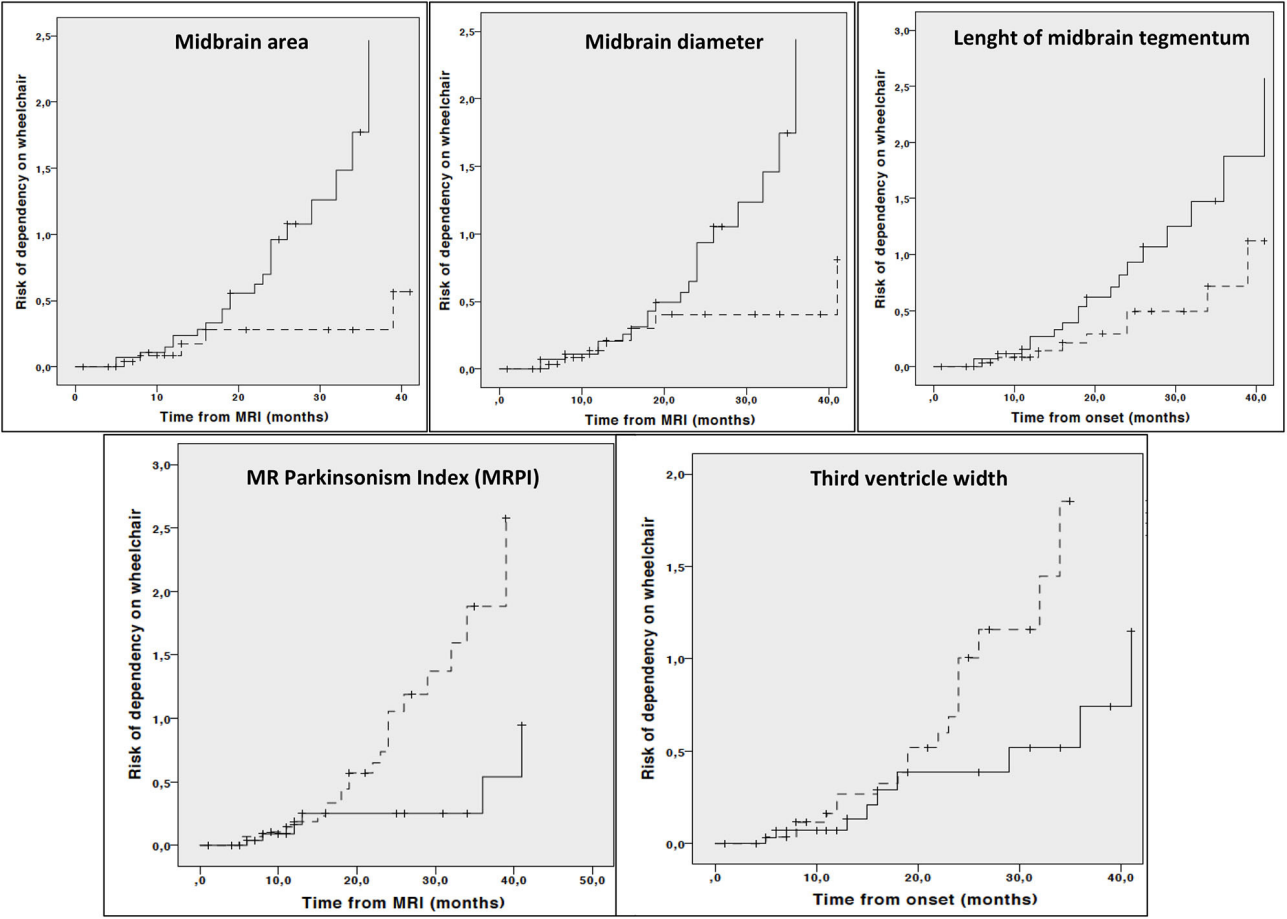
Cumulative risk of dependency on wheelchair by each MRI parameter (less atrophy vs. more atrophy). Only curves with significant log-rank test at 0.05 level are reported. Dotted line: values above median; Continuous line: values below median.
